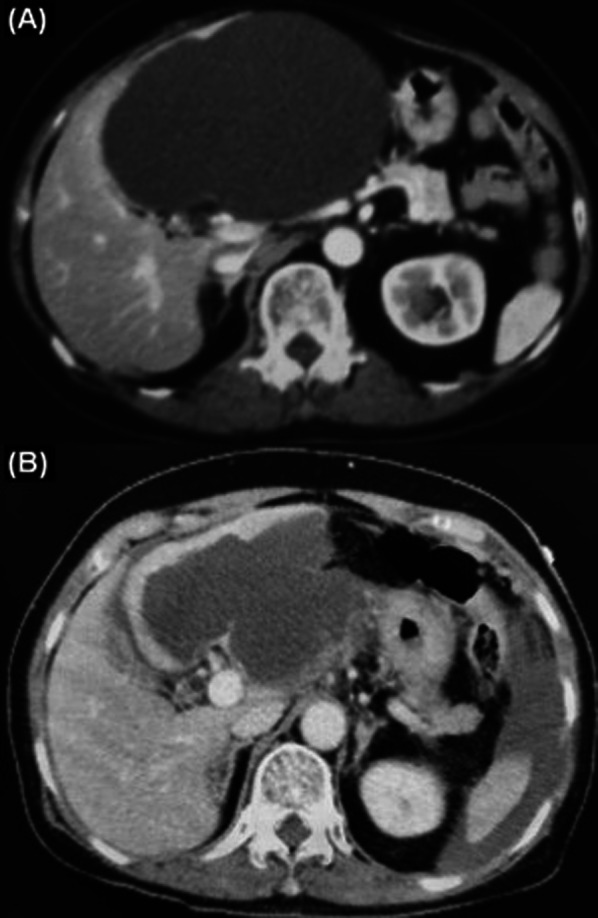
Enhanced CT of case 2. A CT demonstrated a simple hepatic cyst (13 cm) in segment 4 previously. B CT showed ruptured cyst (9.9 cm) and fluid collection between the spleen and the abdominal wall
